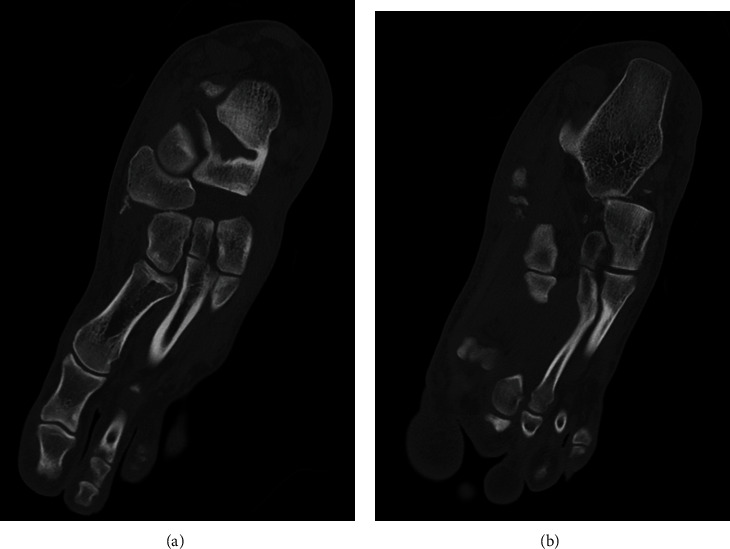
Oblique multiplanar reconstruction CT of the left foot shows dislocation of the (a) naviculocuneiform joint and of the (b) calcaneocuboid joint.
